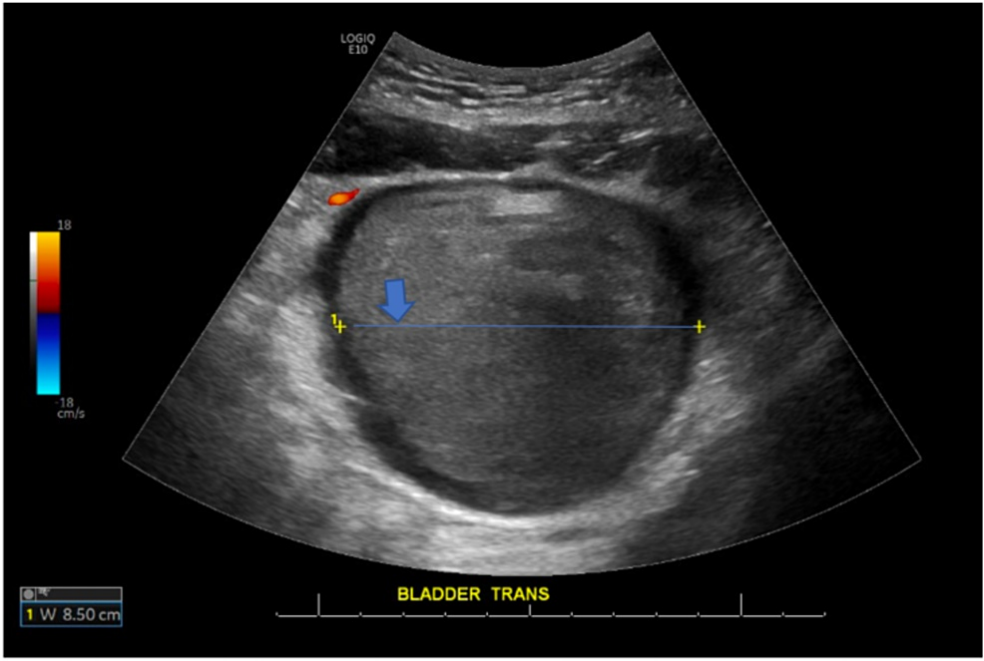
Ultrasound urinary bladder #1: 12.6 x 9.2 x 8.5 cm heterogeneous structure within the urinary bladder likely represent evolving hematoma (blue arrow pointing at the line delineating the width of the hematoma). W: Width; cm: Centimeters.
